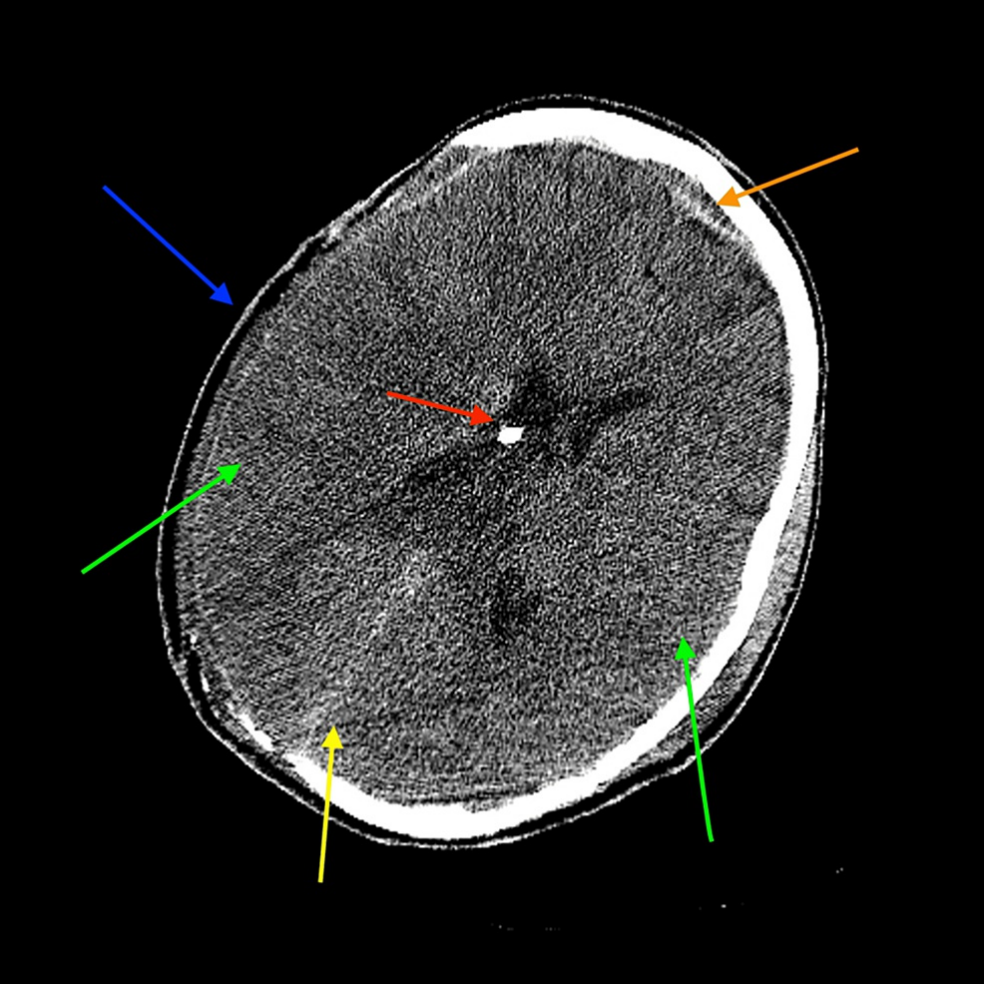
Axial reformat non-contrast CT of the brain at the level of the lateral ventricles. Axial reformat non-contrast CT of the brain at the level of the lateral ventricles demonstrating a right-sided open parietal calvarial defect (blue arrow), diffuse reactive cerebral edema with sulcal effacement (green arrows), right-to-left midline shift, and a partially visualized ventriculostomy catheter in the body of the right lateral ventricle (red arrow). Additionally, there are frontal epidural (orange arrow) and parafalcine blood products (yellow arrow). CT, computed tomography
